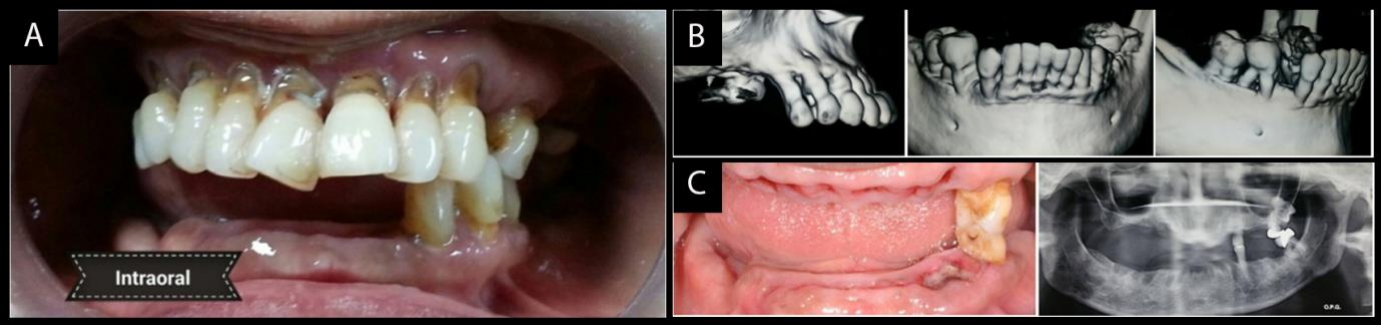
The patient agreed for fixed replacement of teeth and gave the consent for complex reconstruction for implant screw-retained prostheses for both the upper and lower arches. After four months of denture wearing and complete soft tissue healing, the dentures were duplicated in a surgical guide and the patient underwent a CBCT scan to decide optimal positions for implants in each arch. Six tissue-level implants (Myriad Connect, Equinoxmed Belgium) were placed in the mandible between the inter-foramina region (3.3/9.5 mm). The maxilla also had six implants between the maxillary sinus region (3.4/11; 2, 3.4/13; 2, 3.4/9.5;2, Xive Dentsply) (Figures 6 and 7). The retained second premolars of each arch were extracted during the surgery and replaced by implants. The patient had a waiting period of five months for prosthesis fabrication after surgery. The existing complete dentures were adjusted and relined several times to provide a perfect occlusion and oral hygiene was further evaluated every month.
